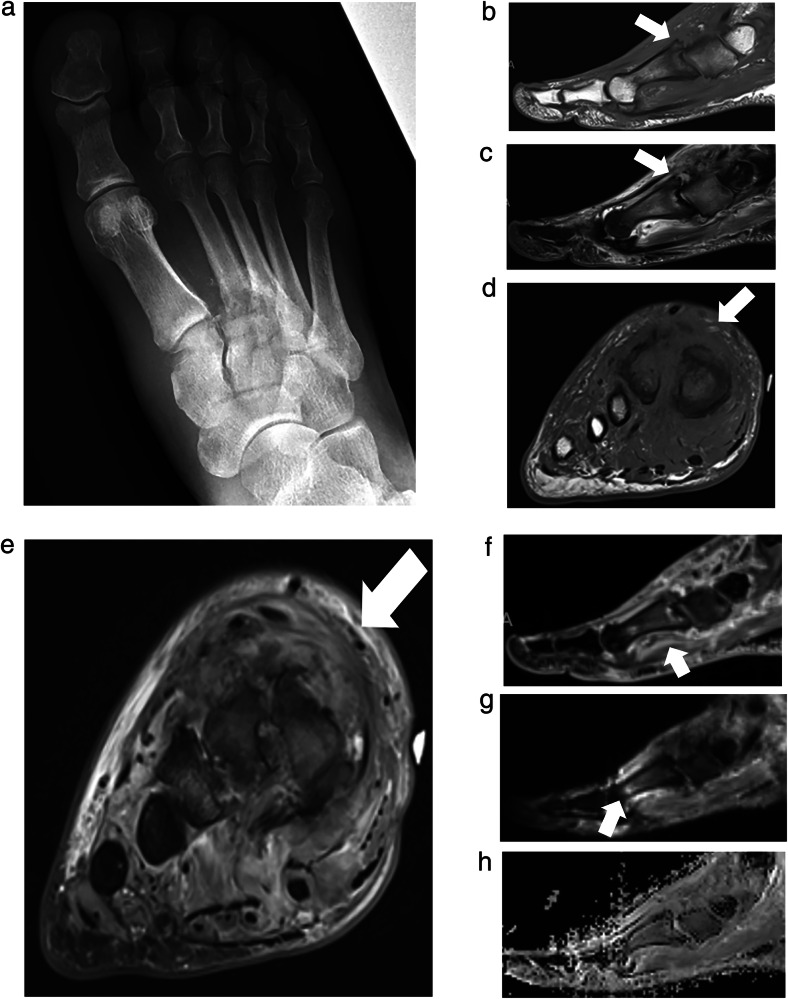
MSKI-RADS NOS. A 54-year-old man with known DM and mid-foot swelling. a Frontal X-ray of the foot. b, c Sagittal T1W and STIR images show 1st tarsometatarsal subchondral erosions and dorsal subluxation with regional soft tissue swelling and edema (arrows). d, e Axial T1W and fsT2W (water map Dixon) images show 1–3rd metatarsal bony cortical erosions and soft tissue swelling (arrows). f Sagittal fsT1W 3D image shows mild bone marrow and diffuse soft tissue enhancement (arrow) without soft tissue ulcer or abscess. g, h Corresponding sagittal DWI and ADC maps show mild T2-shine through of edema of the 1st tarsometatarsal articulation without a restricting abscess (arrow). Findings are consistent with Charcot neuroarthropathy-MSKI-RADS-NOS class
